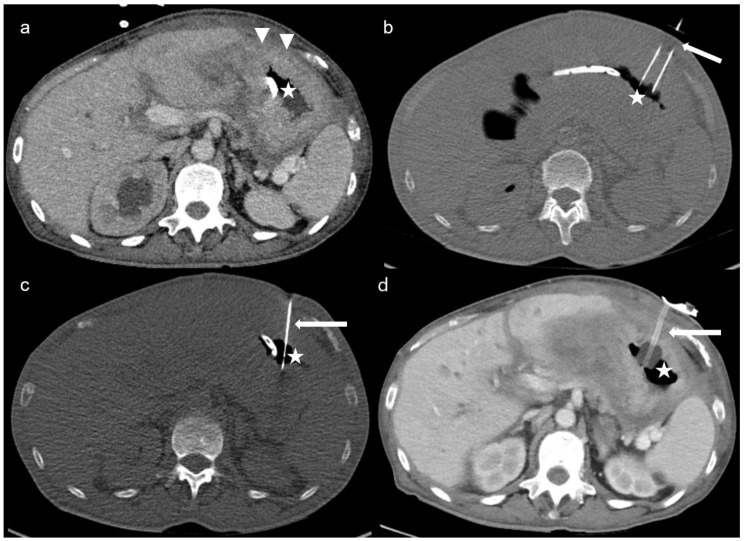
Multiple sequential axial-enhanced CT and unenhanced CT-fluoroscopy images: (a) triangles show thickened peritoneal surfaces due to carcinomatosis, which hinders diaphanoscopy at the endoscopic approach. The nasogastric tube is used to insufflate the stomach *(star)*; (b) the gastropexy device *(arrow)* passes through the abdominal wall and punctions the anterior gastric wall. Gastropexy sutures are applied 2 cm apart to fasten the stomach to the anterior abdominal wall; (c) an 18G access needle *(arrow)* is inserted in the air-filled stomach *(star)*, followed by the introduction of a 0.035″ Amplatz super stiff wire (Boston Scientific, Marlborough, MA, USA) and the insertion of a 16F peel-away sheath (Cook Medical); (d) a 15F gastric tube *(arrow)* is placed in the gastric cavity *(star)* with an inflated balloon (Freka Pexact CH/FR 15).
